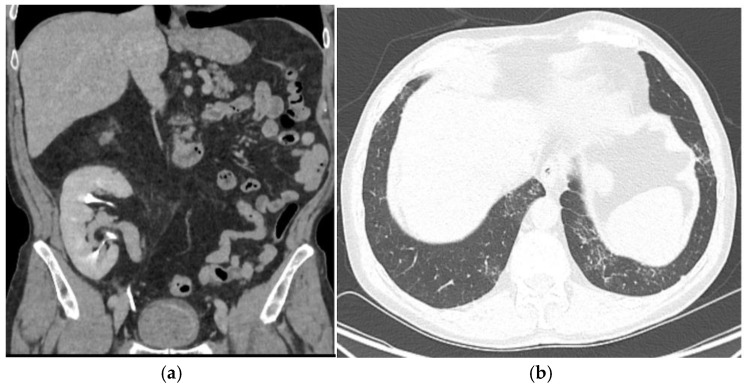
A 1-year follow-up CT scan showing no bladder recurrence, no graft lesion, and no pulmonary metastasis. (a): CT scan showing no bladder recurrence or graft lesion (axial view). (b): CT scan showing no pulmonary metastasis(axial view).
